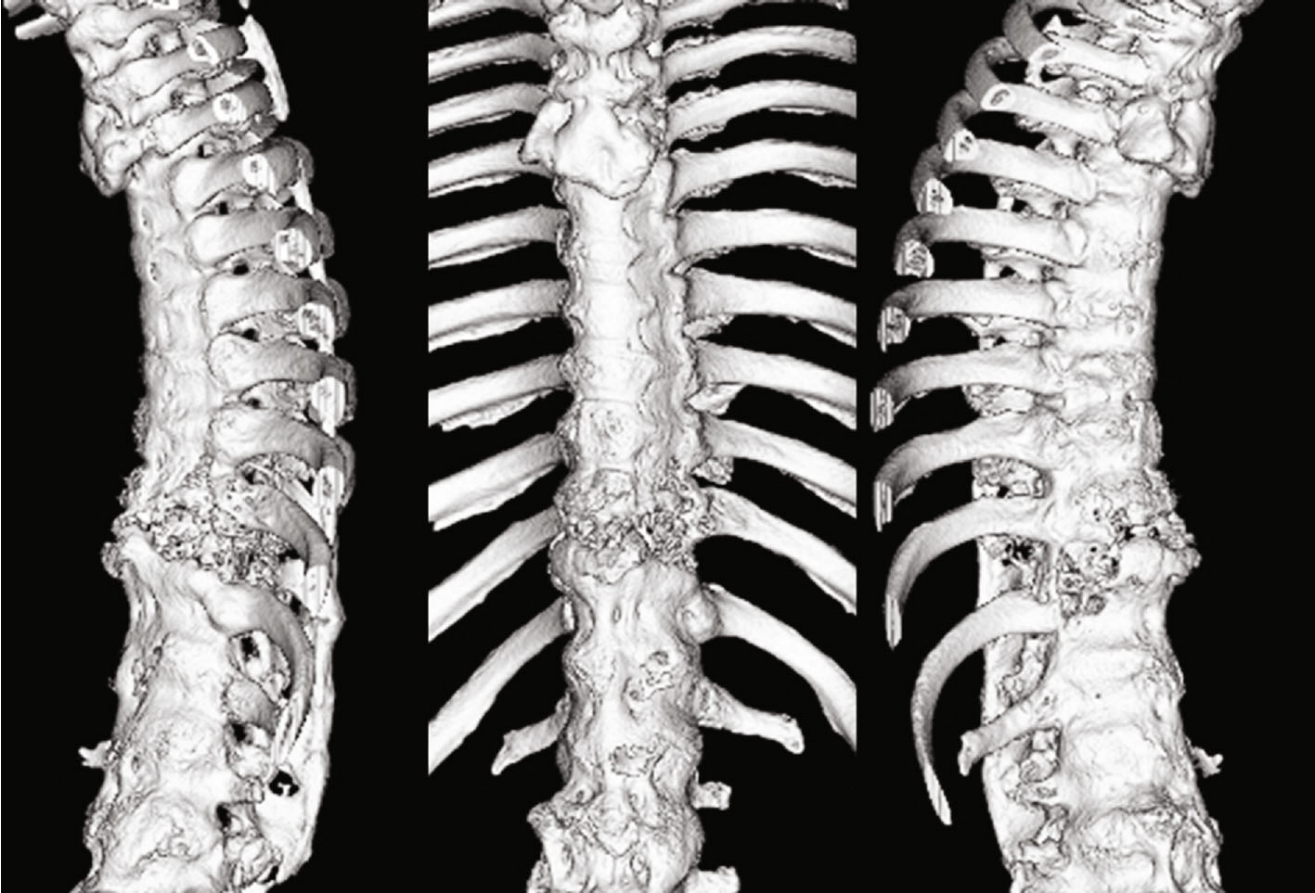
3D CT showing the pathological fracture occurred within the range of DISH, which suggested a hyperinstability.
